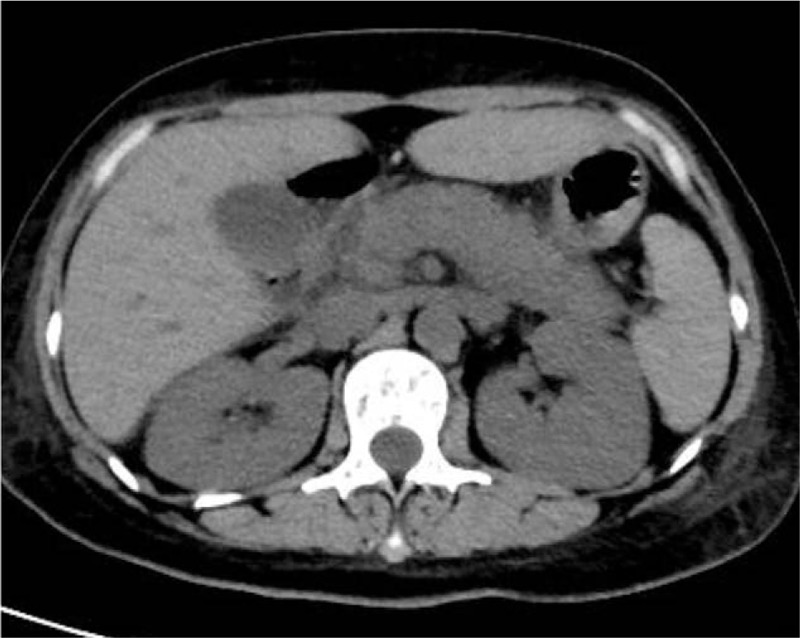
CT of the abdomen showed a swollen pancreas. CT = Computed tomography.
